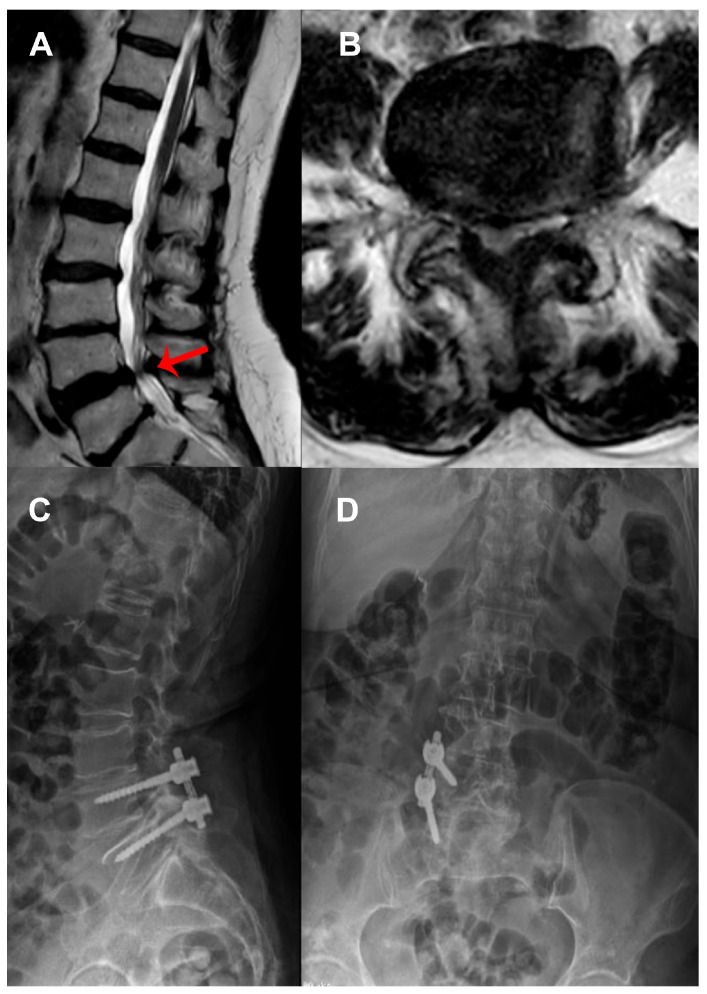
A 71-year-old female patient presented with worsening lower back and posterolateral leg pain, weakness in right ankle dorsiflexion, accompanied by neurogenic claudication after walking 50 m. (A) Sagittal and (B) axial T2-weighted MRI demonstrating spinal stenosis (arrow) at the L4–L5 level. The patient underwent unilateral laminotomy for bilateral decompression and right-sided L4–L5 stabilization using dynamic screws with a helical rod. Postoperative standing radiographs: (C) lateral and (D) anteroposterior views, confirming correct alignment with a dynamic construct.
